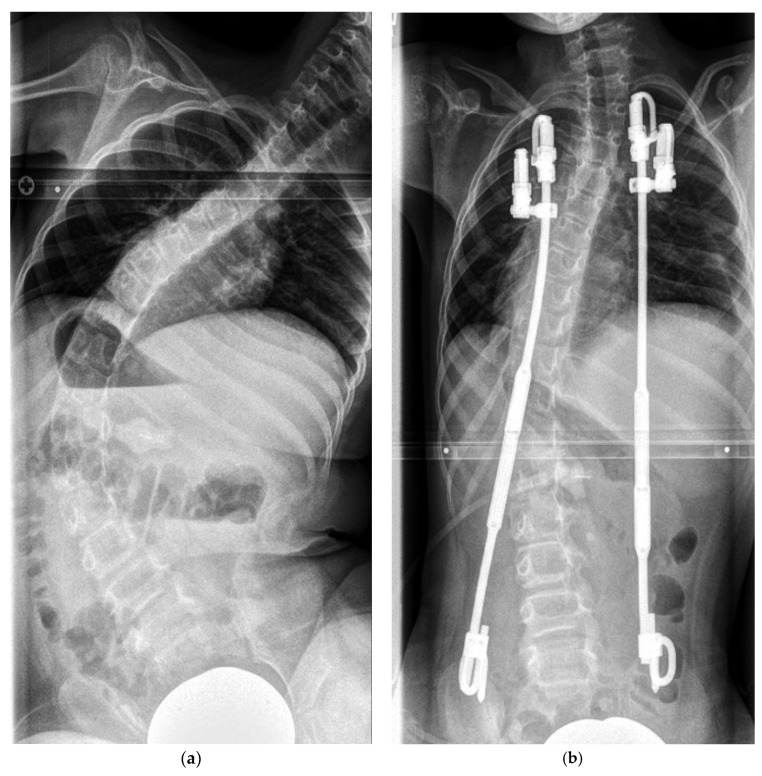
(a) Eight-year-old girl with cerebral palsy. Preoperative X-ray shows a coronal Cobb angle of 94°. (b) Instrumentation with a rib-to-pelvis construct utilizing S-hooks achieved a correction to 31° (Cobb).
